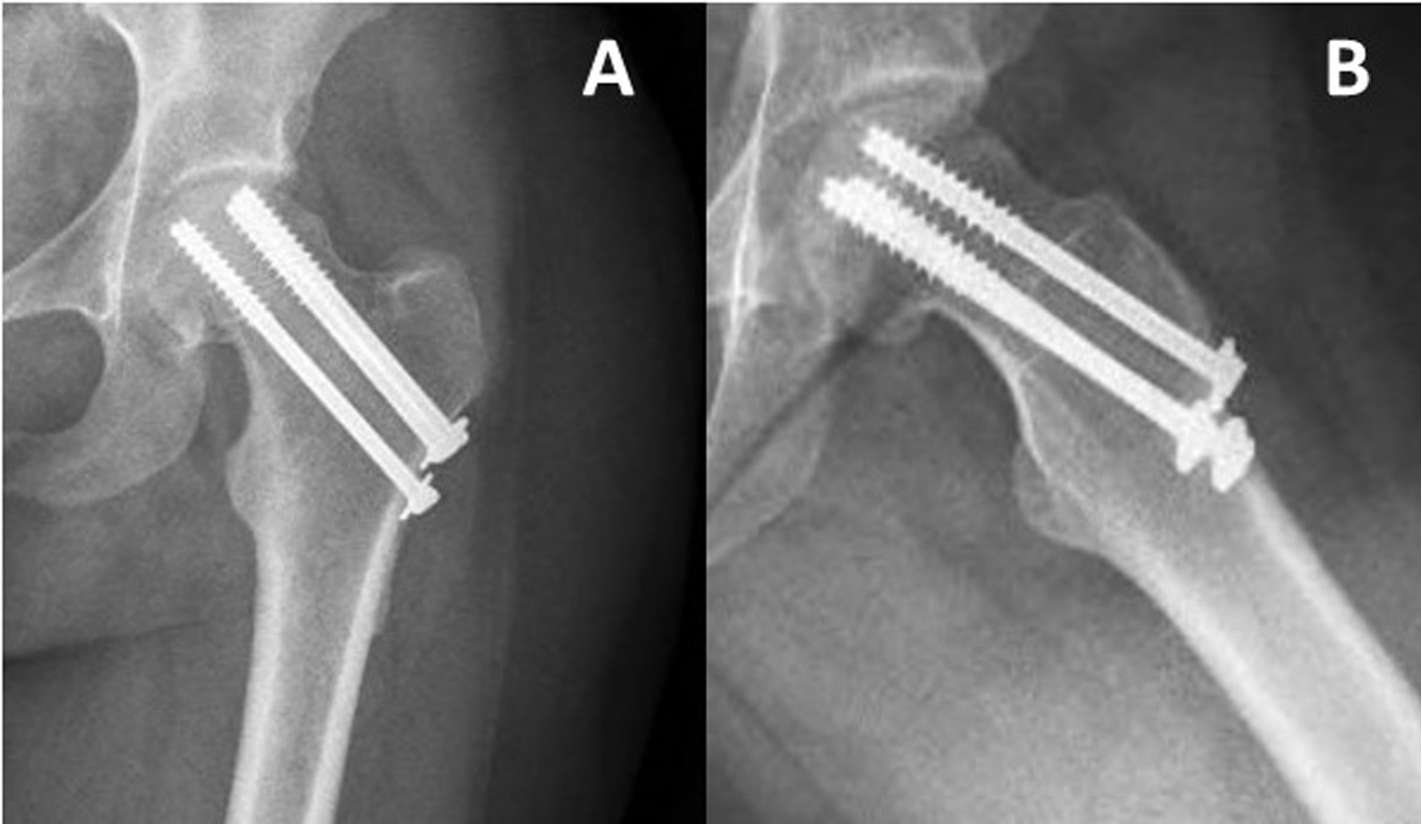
Anteroposterior (A) and lateral (B) radiographs performed on the latest follow-up, 24 months postoperatively, demonstrates consolidation of the fracture with no hardware migration nor intra-articular penetration.
